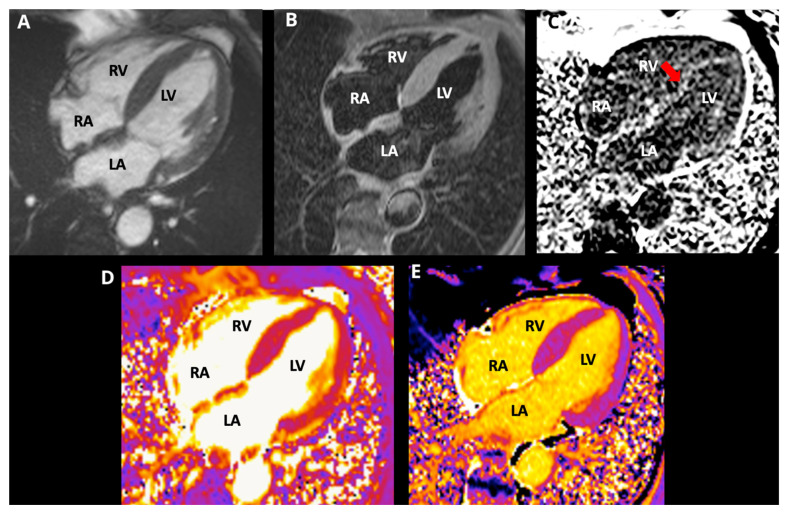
Cardiac magnetic resonance images, 4-chamber view, (A) cine SSFP; (B) T2-STIR, (C) PSIR; (D) T2 mapping; (E) native T1 mapping. CMR revealed symmetric LV thickening (max 17 mm), preserved biventricular systolic function (LVEF 66%, RVEF 54%), difficult myocardial nulling (red arrow), diffuse circumferential subendocardial LGE involving both ventricles with atrial/septal LGE, elevated native T1, and increased ECV (45.5%), consistent with amyloid infiltration. (LA: left atrium, LV: left ventricle, RA: right atrium, RV: right ventricle).
